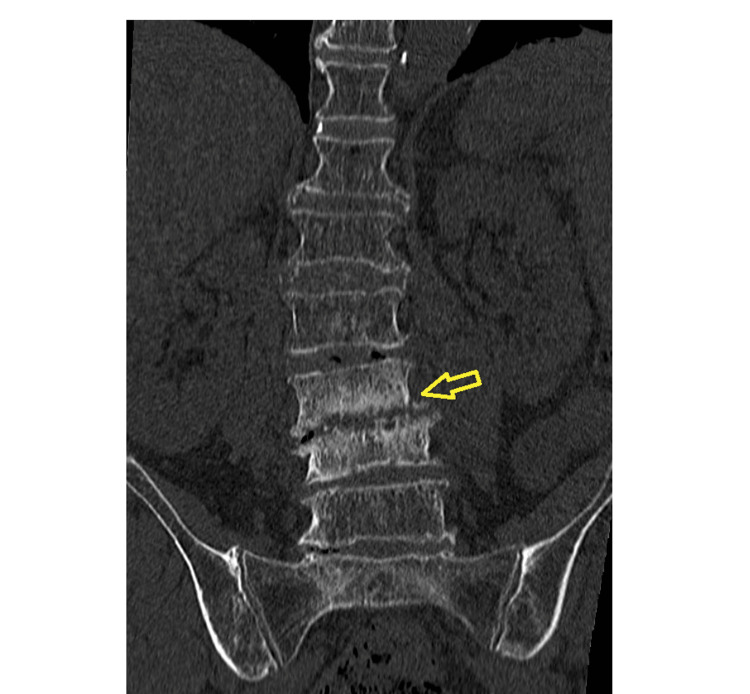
CT of the lumbar spine, coronal view Arrow highlights L4 anterior superior endplate compression fracture with height loss and associated non displaced fractures of the L4 inferior articular processes. Additional findings present for advanced multilevel spondylosis.
